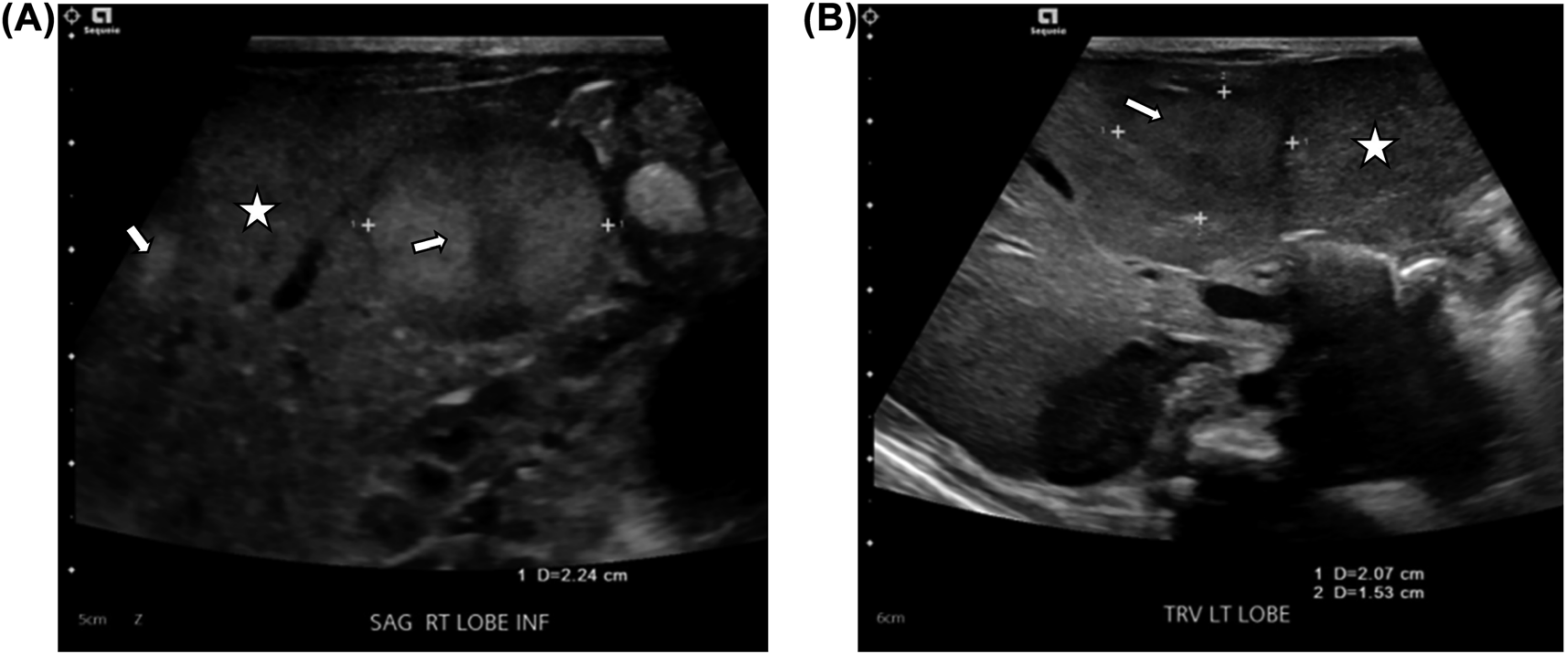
Gray scale sonogram of the liver shows small and large echogenic mass (arrows) on the left and right lobes against the diffusely abnormal hepatic echogenicity (star) background of normal hepatic echotexture.
